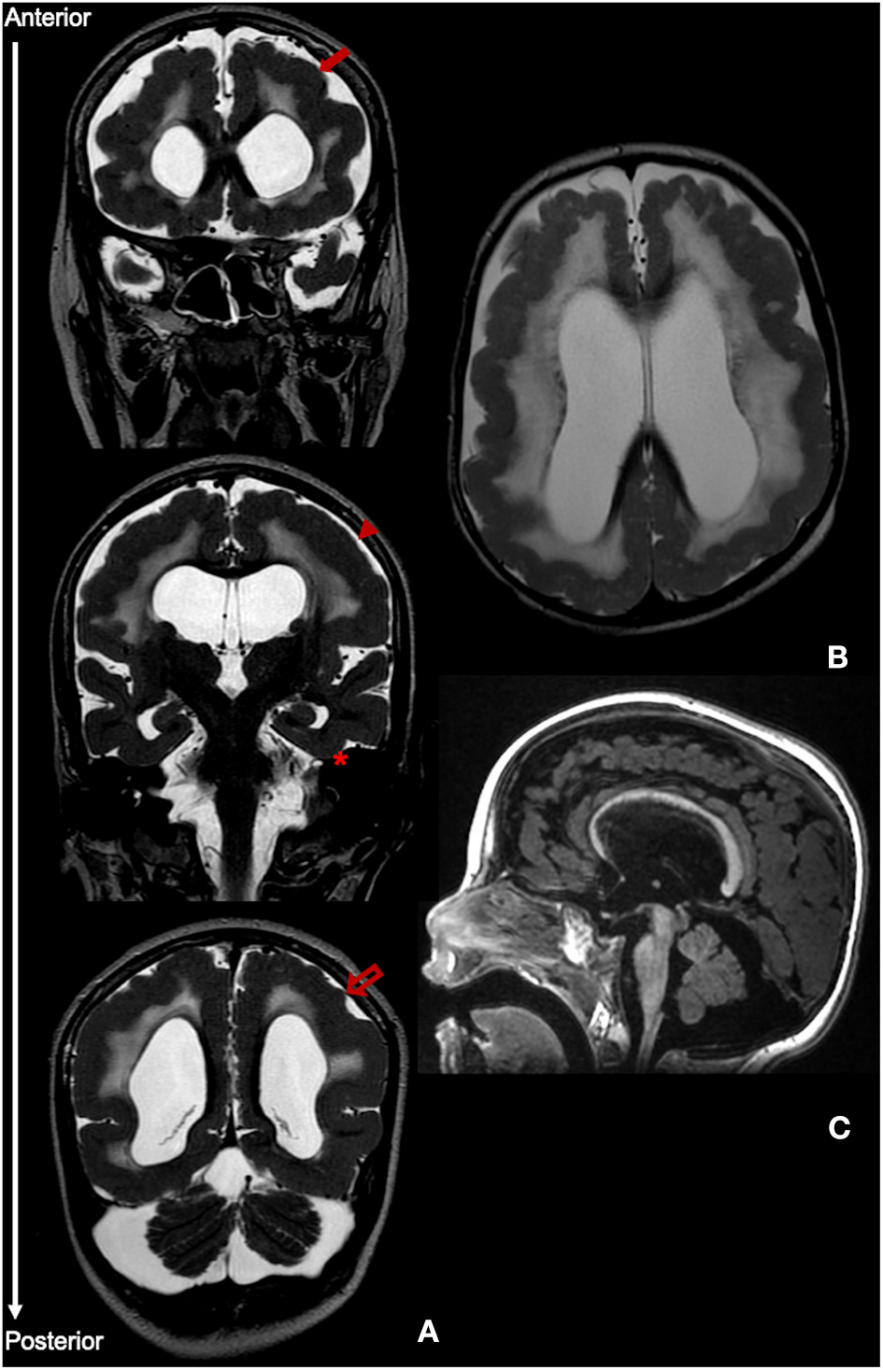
MRI findings at age 11 years. (A) Coronal T2-weighted images showing PMG without an anterior-posterior gradient of involvement, including frontal cortex (closed arrow), parietal cortex (arrowhead) and occipital cortex (open arrow). There is relative sparing of the temporal lobes (asterisk). (B) Axial T2 image displaying diffuse white matter hypomyelination. (C) Sagittal T1 image highlighting pontine and cerebellar hypoplasia.
